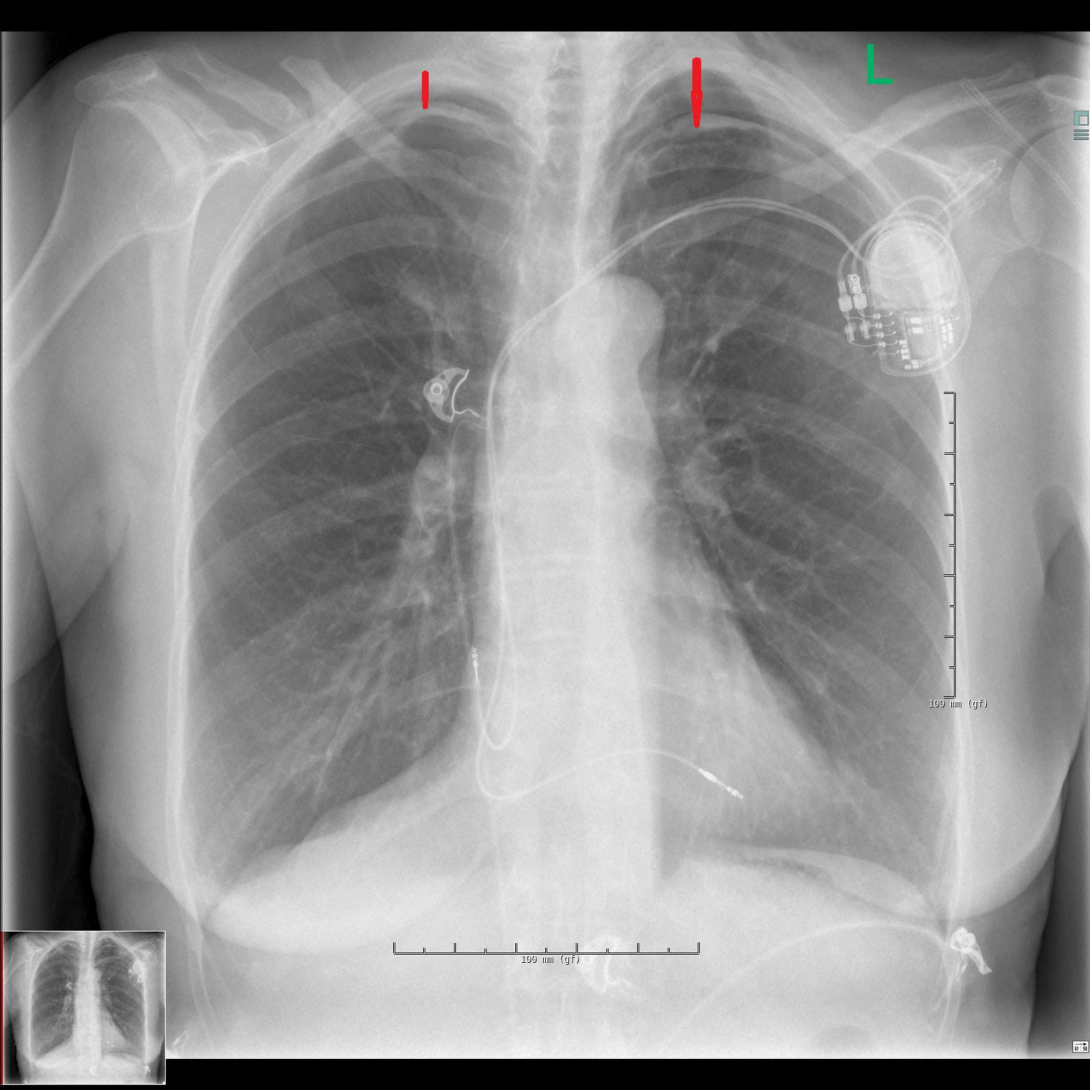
Chest X-ray shows bilateral apical pneumothorax (red arrows) 3 hours after implantation of the pacemaker (day 1).
